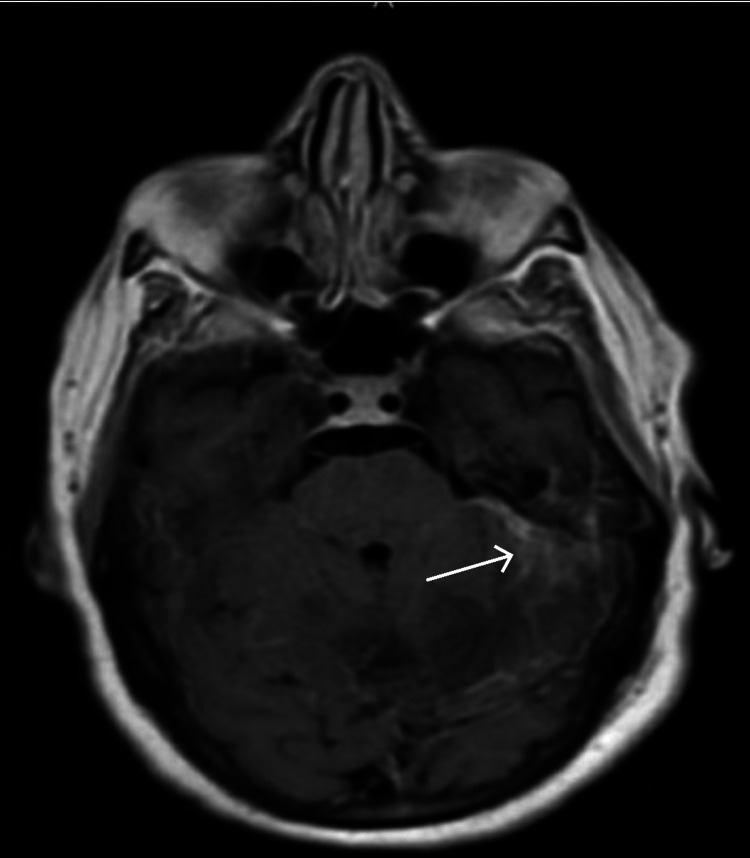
MRI brain showing left posterior fossa epidural abscess on T1 sequence MRI: Magnetic resonance imaging
